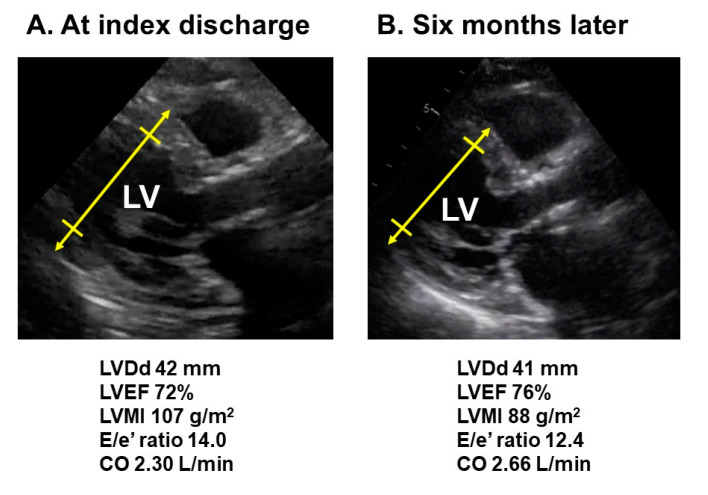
Long-axis views of transthoracic echocardiography obtained at index discharge (A) and six-month follow-up (B); LV, left ventricle; LVDd, left ventricular end-diastolic diameter; LVEF, left ventricular ejection fraction; LVMI, left ventricular mass index; CO, cardiac output.
